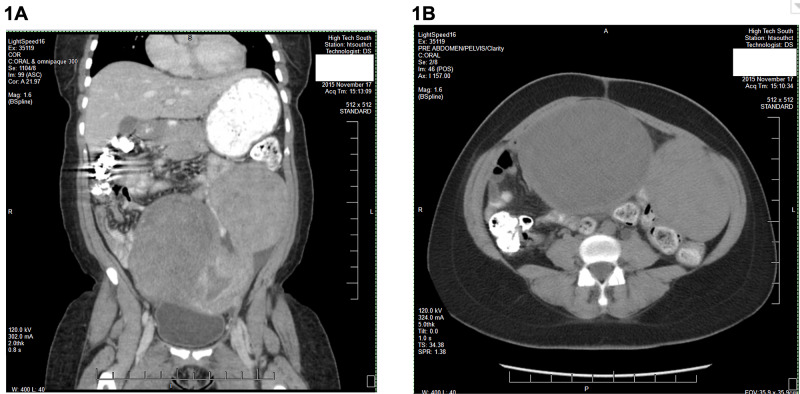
Pelvic CT showing massive fibroid uterus measuring 19 cm in length and 15 cm in width with one large subserosal fibroid projecting superiorly to the right measuring 13 x 12 cm, another projecting superiorly to the left measuring 10 x 8 cm, evidenced by coronal view (A) and again by transverse view (B).
